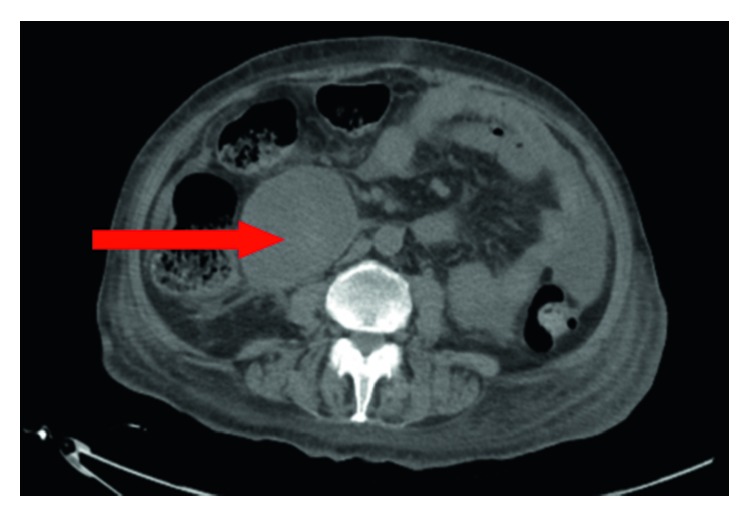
CT scan of the abdomen showing the mass near the pancreatic head.
